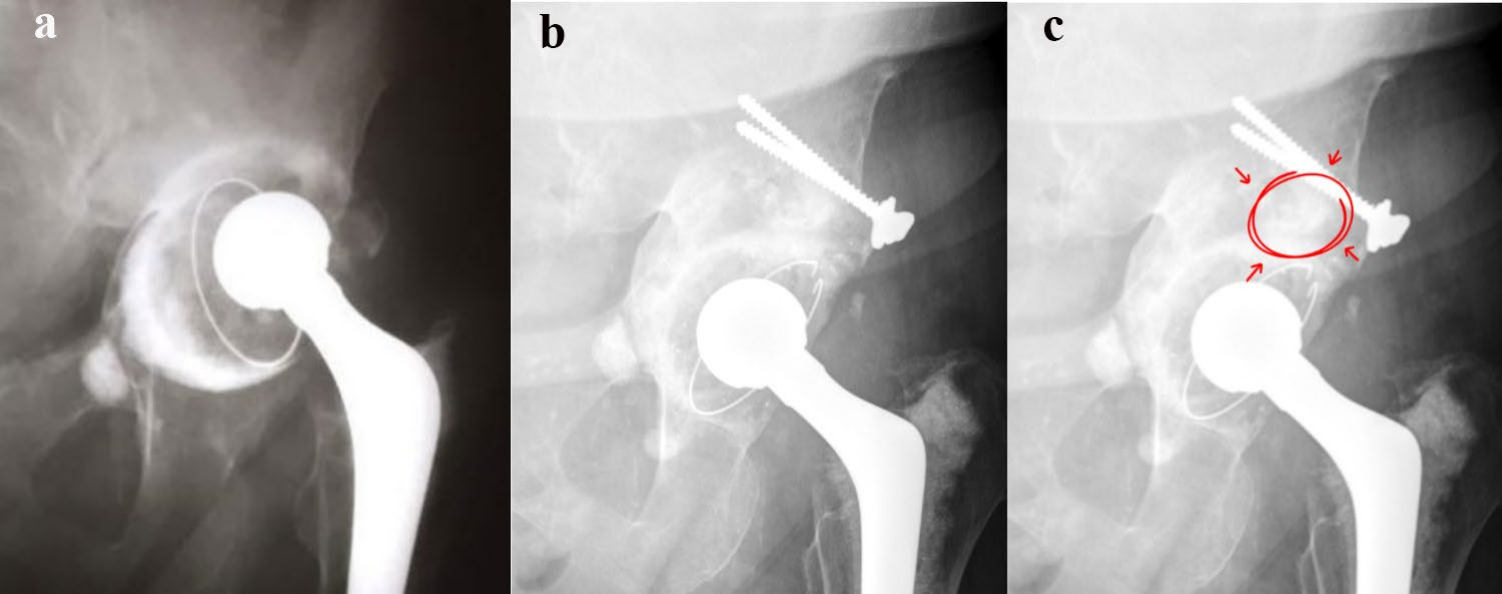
A Paprosky type 2B acetabular defect; B postoperative radiograph at 68 months follow-up. C Containment hole signalized in red. Source: the Authors
